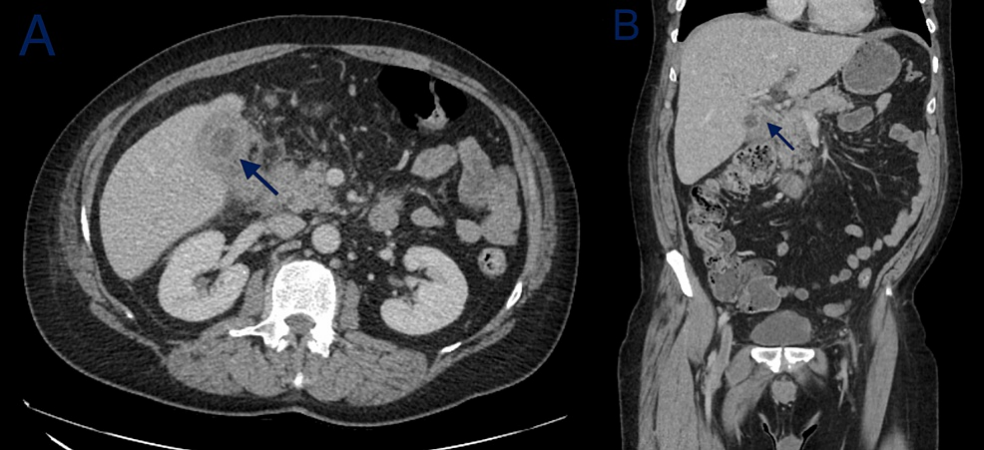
Contrast-enhanced CT images of the abdomen and pelvis (A) Axial and (B) coronal images showing extensive inflammation and diffuse moderate gallbladder wall thickening, with an extraluminal collection of fluid. There is effacement of pericholecystic fat with no calcified gallstones. Proximal common bile duct enhancement adjacent to inflamed gallbladder. Mildly enlarged lymph nodes in the gastrohepatic ligament.
